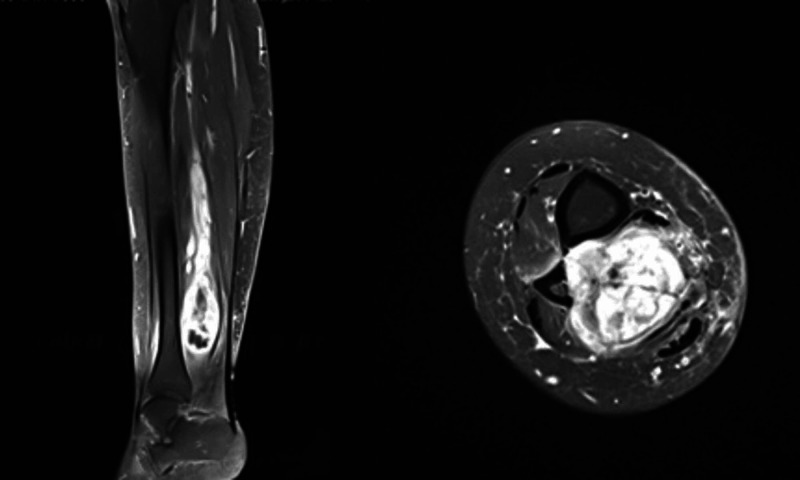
Sagittal and axial MRI views of the mass
